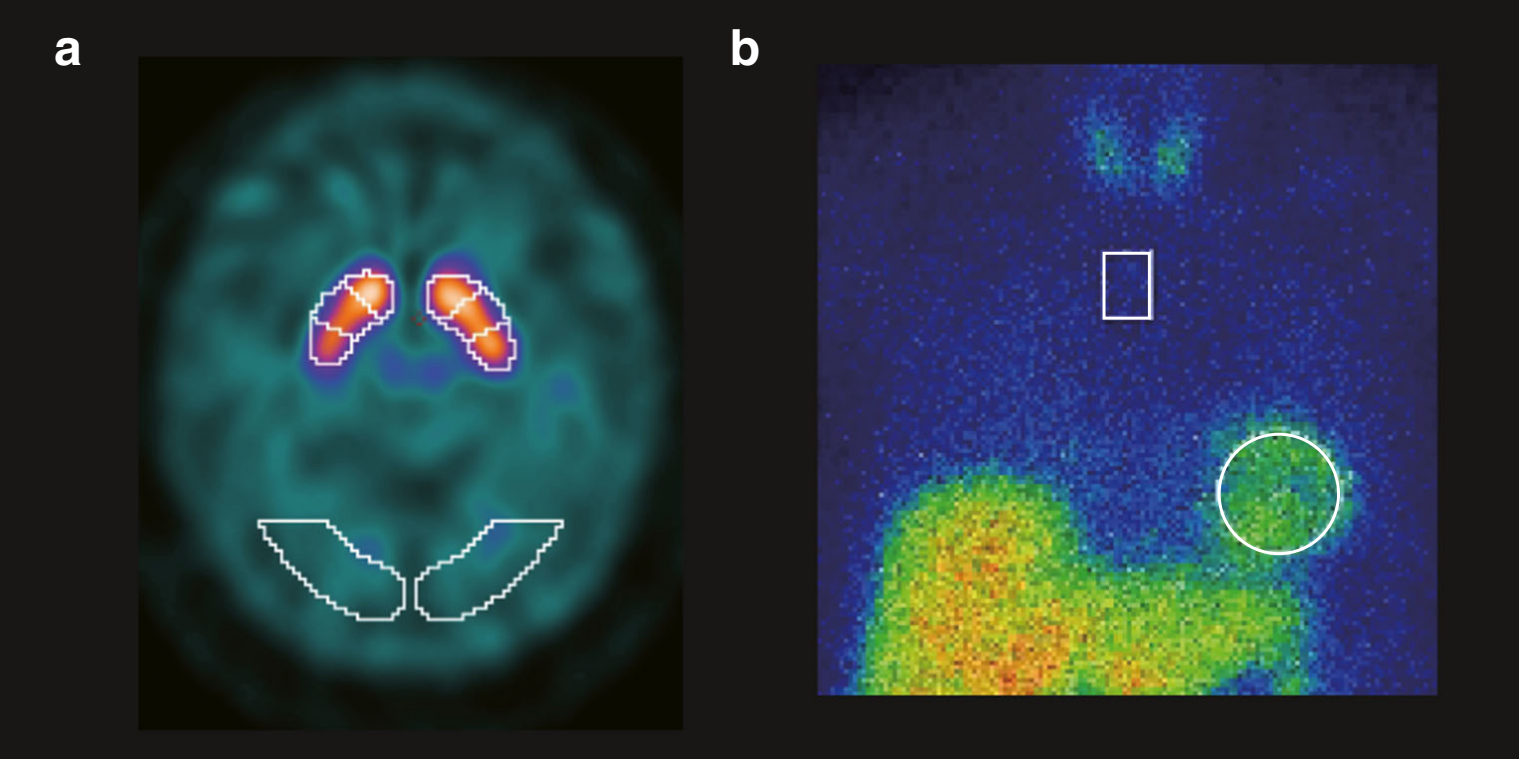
Representative DAT SPECT and MIBG scintigraphy images. a A representative VOI setting of DAT SPECT. A commercially available software package DaTQUANT was used. b A representative ROI setting of MIBG scintigraphy. A commercially available software package smartMIBG was used. Abbreviations: *DAT* dopamine transporter, *MIBG*
